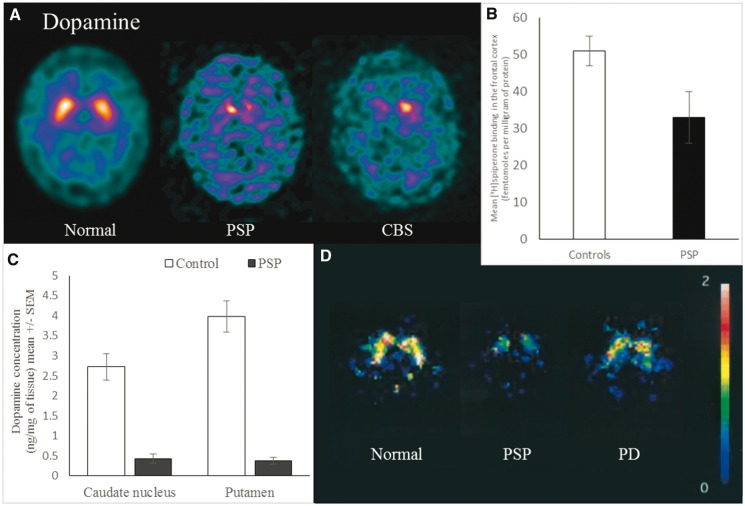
Dopamine deficits in PSP and CBS. (A) Ioflupane SPECT scan showing reduced pre-synaptic dopaminergic neurons in the striatum of PSP and CBS compared to a normal scan. (B) Post-mortem dopamine receptor levels (measured by spiperone binding) are reduced in the frontal cortex in PSP. Graph of data from Ruberg *et al.* (1985). Reprinted with permission from Wiley. (C) Dopamine levels are reduced in the caudate nucleus and putamen in PSP. Graph of data from Ruberg *et al.* (1985). (D) D2 dopamine receptor levels (measured by ^123^I-iodobenzofuran SPECT) are reduced in the striatum of PSP when compared with healthy controls and Parkinson’s disease. From Oyanagi (2002). Reprinted with permission from Wiley.
